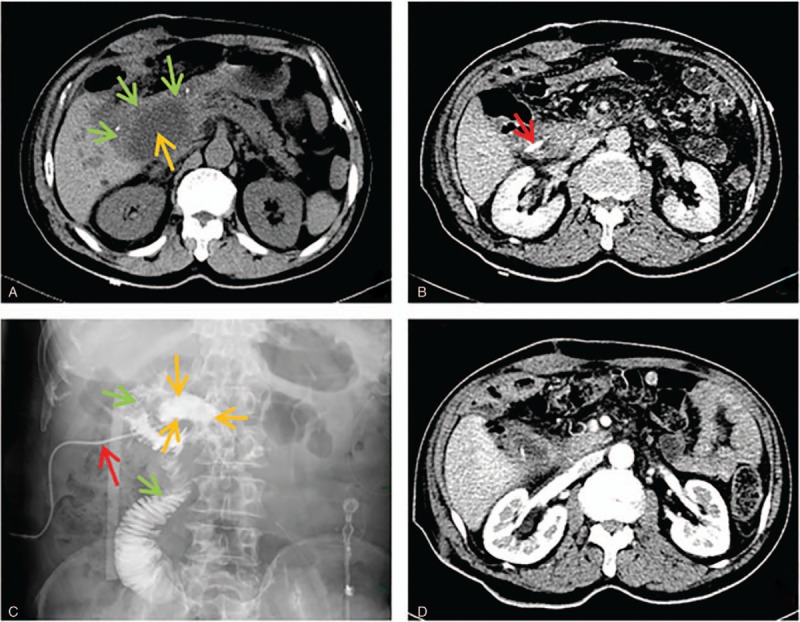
Transintestinal afferent loop approach. (A) Axial CT scans showed that an abscess (light orange arrow) with size of 5 × 6 cm was detected in previous region of pancreatic head and was surrounded by intestinal afferent loop (green arrow). (B) Axial CT scans obtained 3 days after the placement of drainage catheter showed that the tip of catheter (red arrow) was inside abscess cavity. (C) Drainage catheter pyrography obtained 1 week after the procedure confirmed that the drainage catheter (red arrow) got through the jejunum (green arrow) into the abscess cavity (light orange arrow). (D) Axial CT scans 10 days after removal of drainage catheter showed that abscess cavity completely collapsed.
